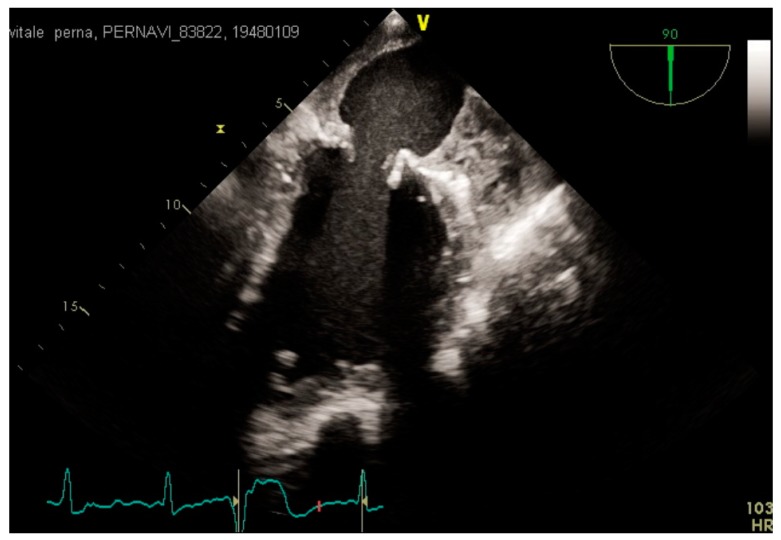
Transesophageal echocardiogram (TOE) intercommissural view showing massive left atrium (LA) and left atrial appendage (LAA) thrombosis.
